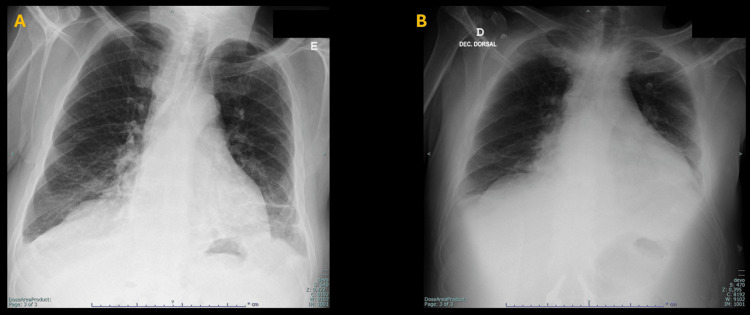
There is a two-day interval between chest X-rays A and B from the same patient. Image panel A represents an orthostatic posteroanterior chest X-ray that reveals a subtle increase in the cardiac silhouette and mild costophrenic sinus effacement bilaterally. Image panel B is a decubitus anteroposterior chest X-ray obtained two days after the chest image in panel A, and demonstrates significant progression, with a marked increase in the cardiac silhouette and more pronounced bilateral costophrenic sinus effacement.
